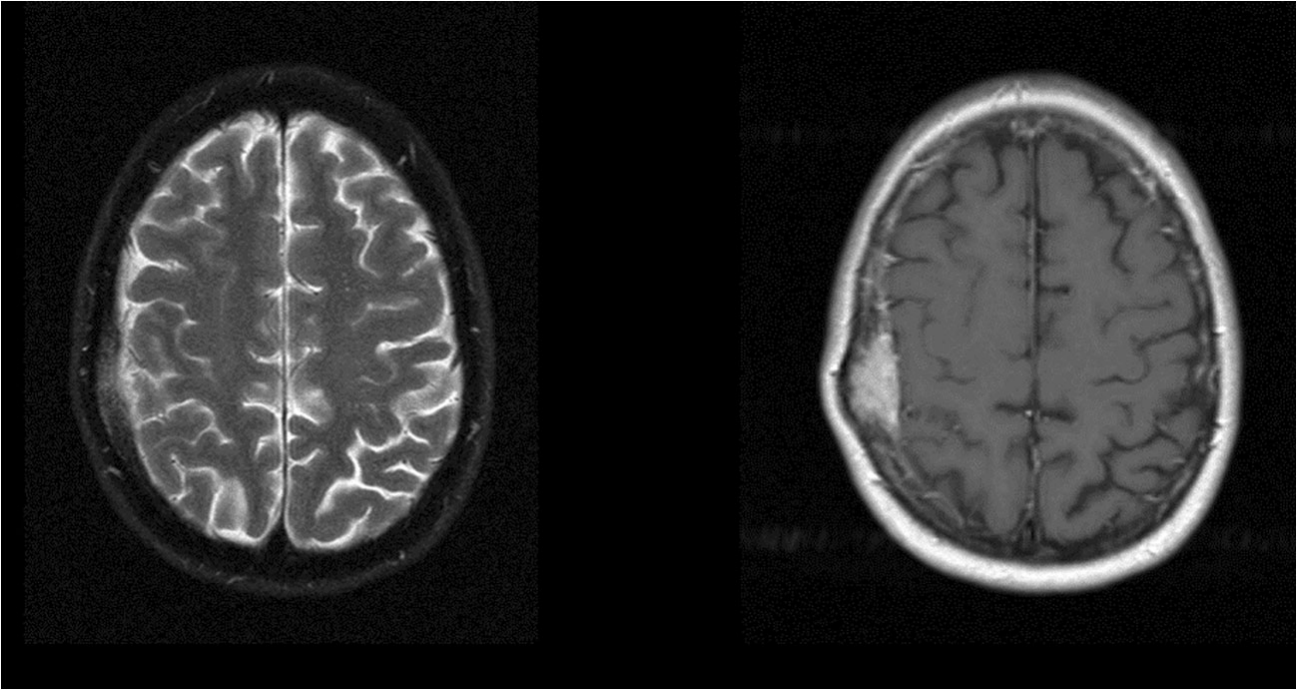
Magnetic resonance images. Meningioma without edema. Left image T2-weighted image; right image T1-weighted image with gadolinium
